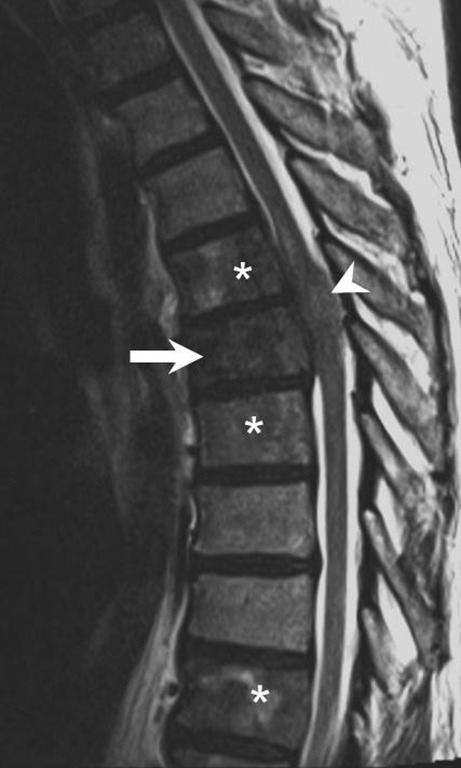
Sagittal T2-weighted images show patchy heterogeneous signal intensities in the vertebral body of Th5 (*arrow*) as well as within various other vertebral bodies of the thoracic spine (*asterisks*). An intraspinal, perimedullary lesion was found that showed isointense signal when compared to the myelon (*arrowhead*)
